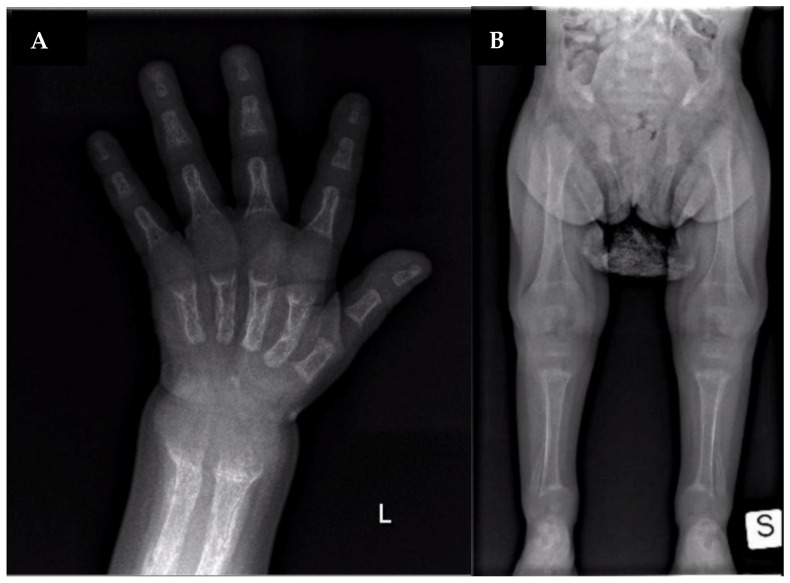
X-ray at diagnosis. (A): lateral widening of metaphyseal region; bone demineralization; fractures of metacarpus, radio, and ulna. (B): lateral widening of metaphyseal region; bowed fibula and tibia; bone demineralization; fractures of femur and fibula.
